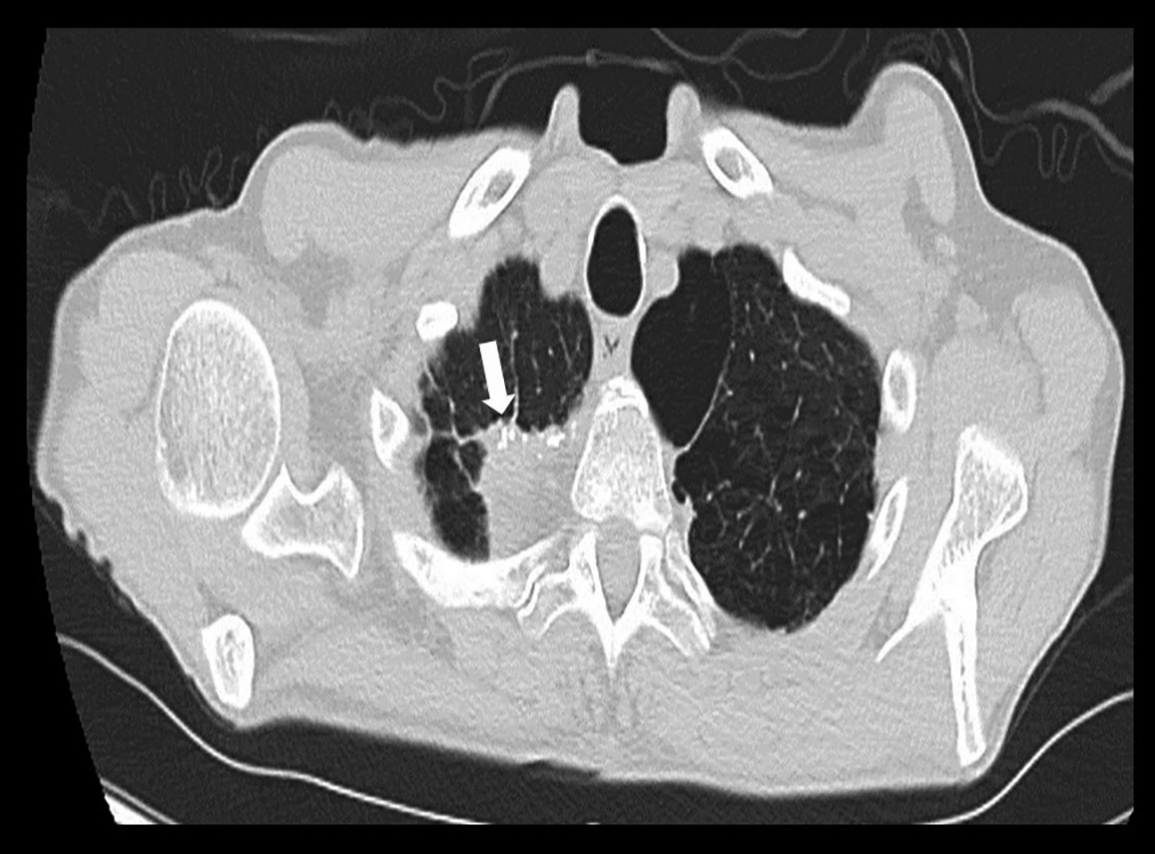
CT chest without contrast (axial plane) showing showed a 2.9 cm right apical mass concerning for metastatic disease (arrow).
